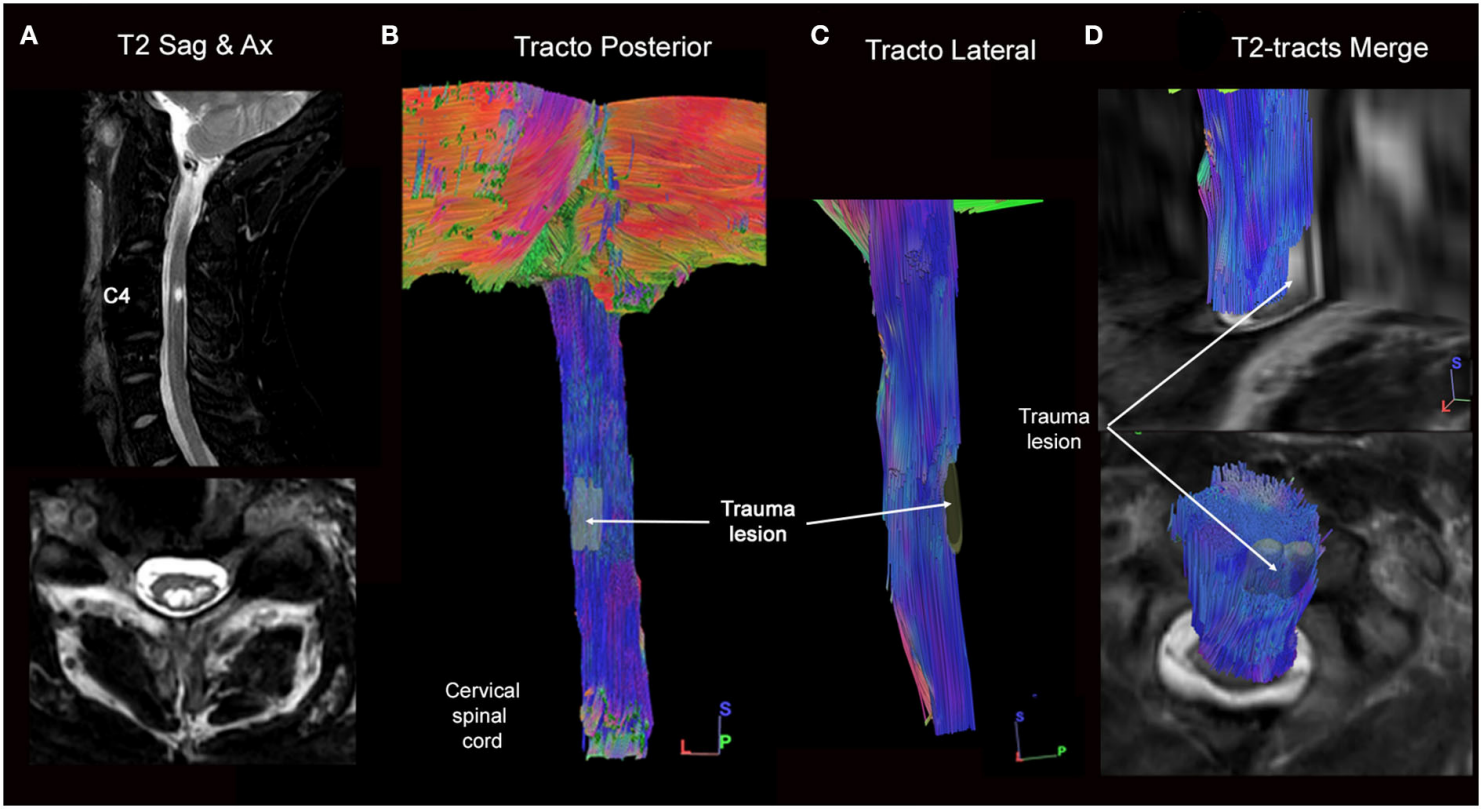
Full cervical cord tractography in a patient with spinal cord injury. (A) Sagittal and axial T2-weighed images showing intramedullary hypersignal at the C3–C4 spine level. On an axial image, intramedullary T2-hypersignal is seen on the left dorso lateral part of the spinal cord. (B) Dorsal and (C) lateral views of spinal cord tractography showing the complete interruption of left dorso-lateral spinal cord fibers. (D) Overlay of the spinal cord tractography on the sagittal T2-weighted MR images, where the correspondence between T2 hypersignal and fibers loss was perfectly defined.
